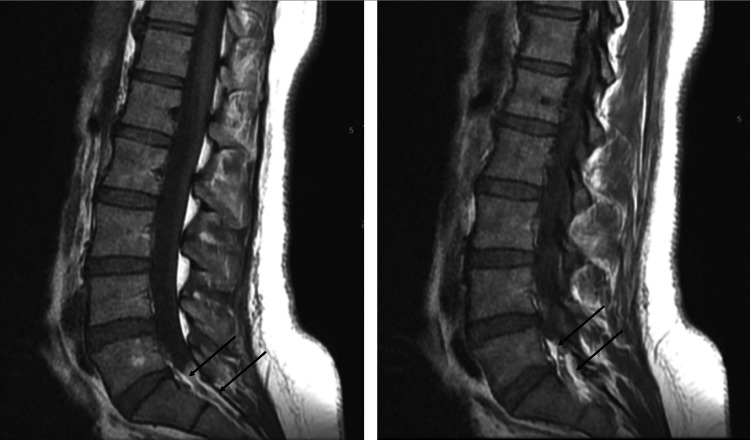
MRI (sagittal T1-weighted) of the lumbosacral spine revealed thecal sac narrowing at the L5 level, with complete effacement of the thecal sac at the S1 and S2 levels. These findings are consistent with epidural lipomatosis following a steroid injection.
